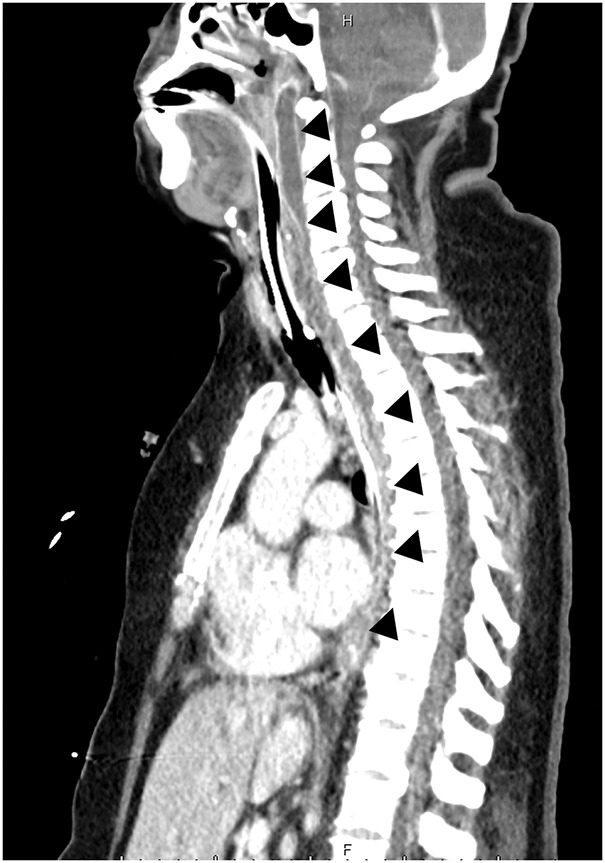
Enhanced CT scan of a sagittal plane before emergency surgery. Fluid collection with an enhanced wall, indicating an abscess in the hypopharynx extending to the mediastinum (black triangles).
